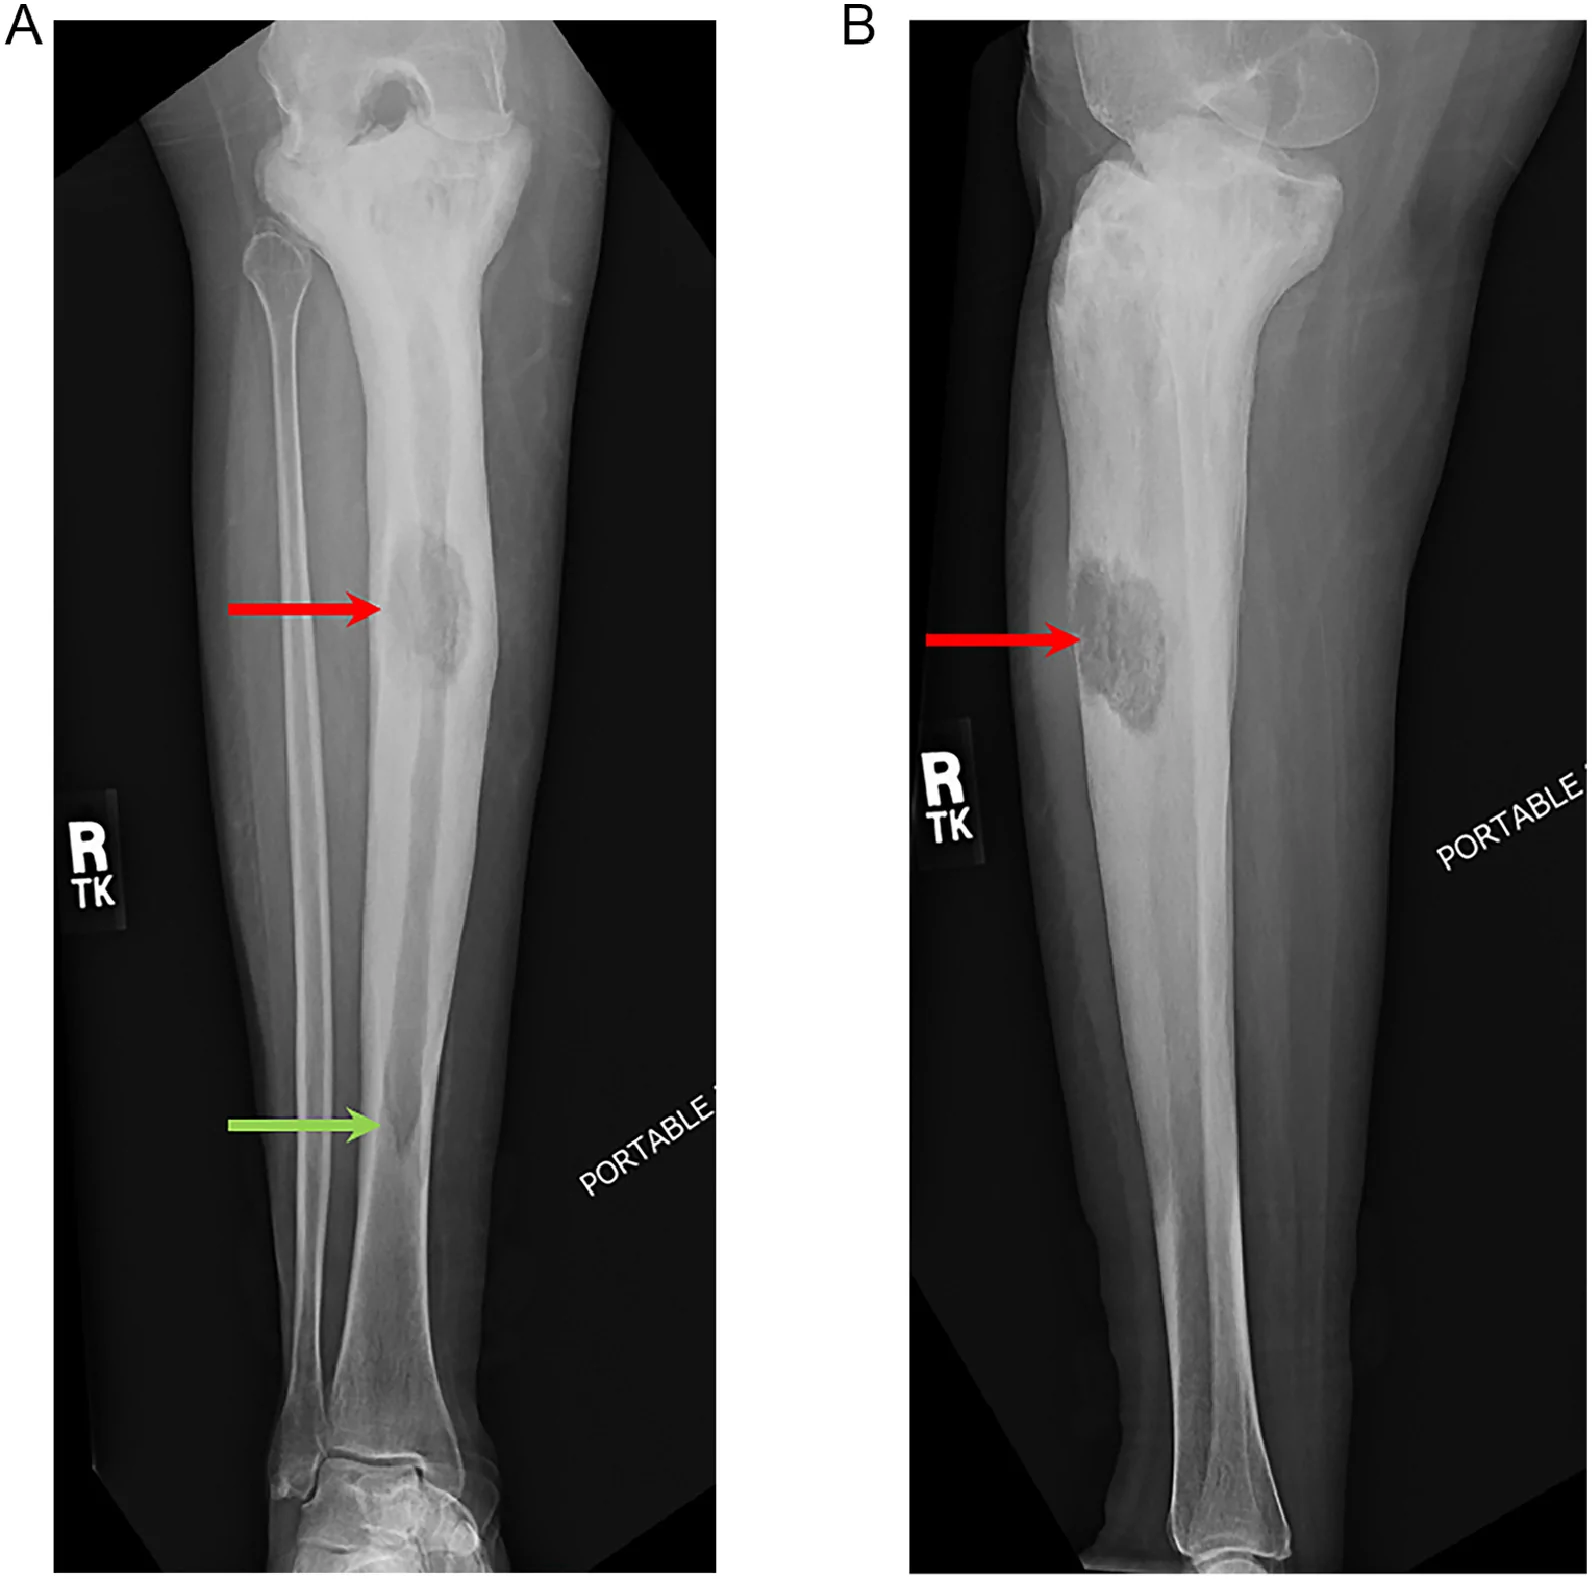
AP (A) and lateral (B) radiographs of the right tibia/fibula demonstrate extensive Paget’s disease of the tibia with demarcation indicated by the tapered lucency (“blade of grass” sign) at the distal tibial diaphysis (green arrow). Compared to Fig. 1, Fig. 2, there is a new lytic lesion in the proximal to mid tibial diaphysis with cortical thinning and discontinuity anteriorly (red arrow). No osteoid matrix is seen in the lesion.
